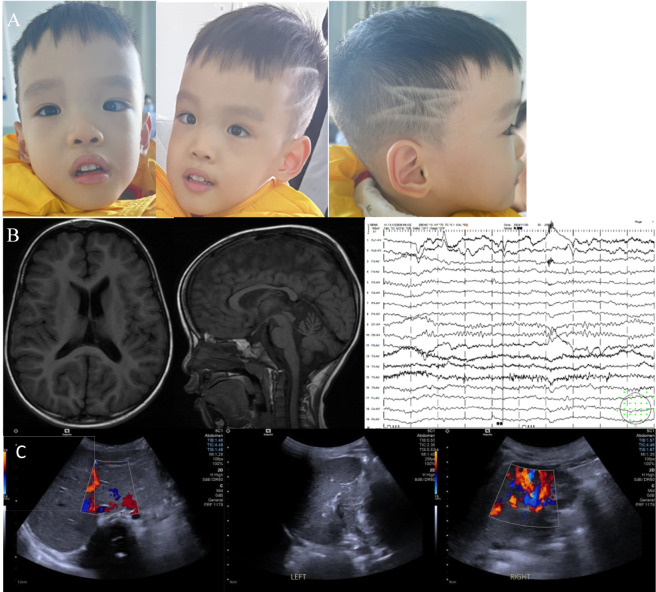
Clinical and imaging findings of the patient. (A) Facial photographs at 3 years of age showing an elongated face, midface hypoplasia, frontal bossing, hypertelorism, arched eyebrows, low-set ears, esotropia, and scaphocephaly. Head circumference was 37 cm (<third percentile). (B) Left and middle panels: Electroencephalogram showing normal background activity. Right panel: Non-contrast brain MRI at 3 years of age revealing no significant abnormal signal in the bilateral frontal, parietal, temporal, or occipital lobes. No widening of sulci, fissures, or cisterns was observed, and the ventricular system was not enlarged. The cerebellum and brainstem appeared normal. (C) Left and middle panels: Abdominal ultrasound at 4 years of age showing normal liver morphology. Right panel: Renal ultrasound demonstrated bilaterally reduced renal longitudinal diameters compared with age-matched norms. Left kidney: 6.0 cm × 2.5 cm (anteroposterior diameter) × 2.7 cm; right kidney: 6.0 cm × 2.6 cm (anteroposterior diameter) × 2.5 cm.
